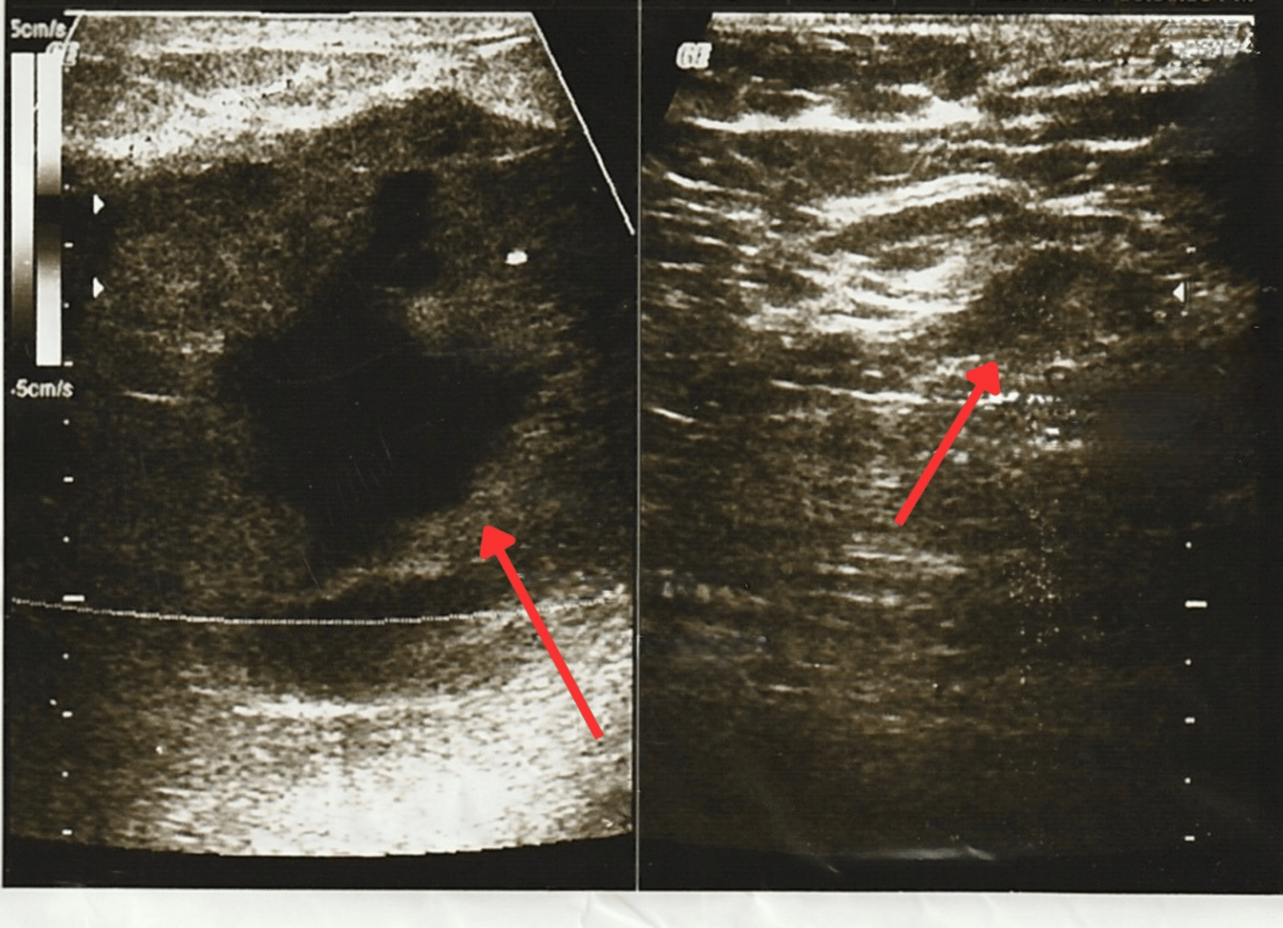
New breast ultrasound where an oval mass with central cystic degeneration and vascularity can be observed First image arrow: Retroareolar mass and vascularity Second image arrow: Oval mass with central cystic degeneration
